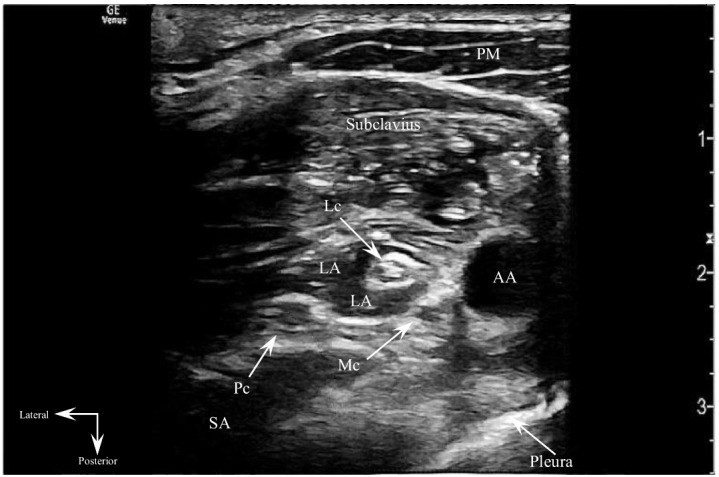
Transverse sonogram post-costoclavicular brachial plexus block performance. Anechoic local anesthetic injectate is visualized enveloping and spreading apart the various costoclavicular brachial plexus cords. *AA*, axillary artery; *LA*, local anesthetic; *Lc*, lateral cord; *Mc*, medial cord; *Pc*, posterior cord; *PM*, pectoralis major; *SA*, serratus anterior.
